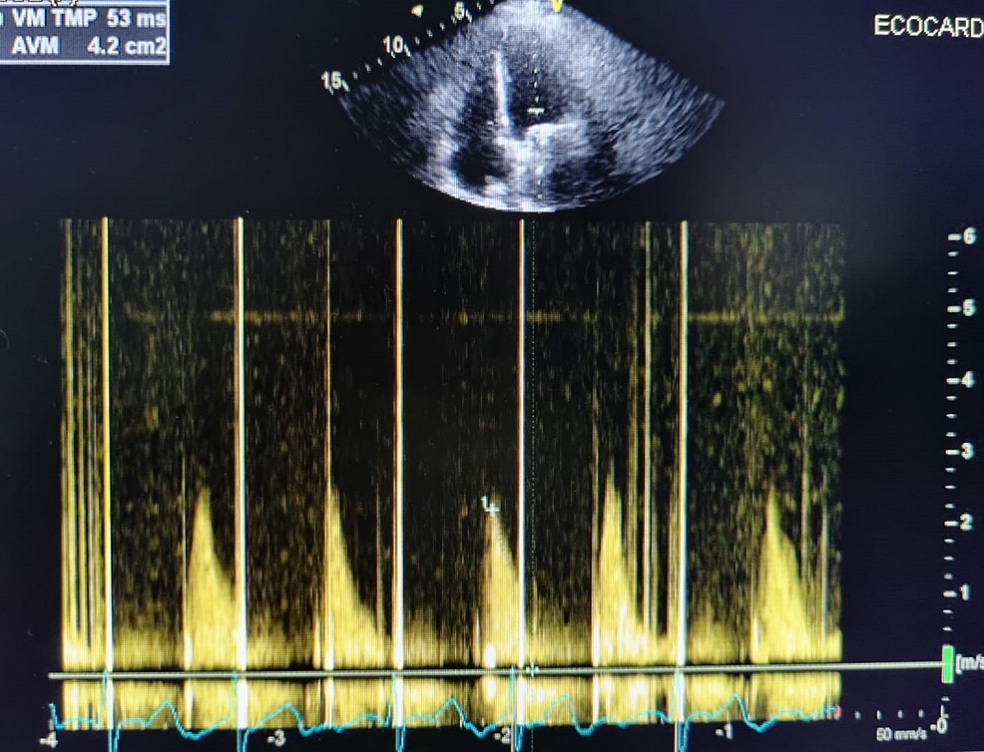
Color Doppler echocardiogram After two months, a transthoracic ultrasound showed that normofunctional mitral prosthesis is found with no evidence of thrombi or intracavitary masses.
